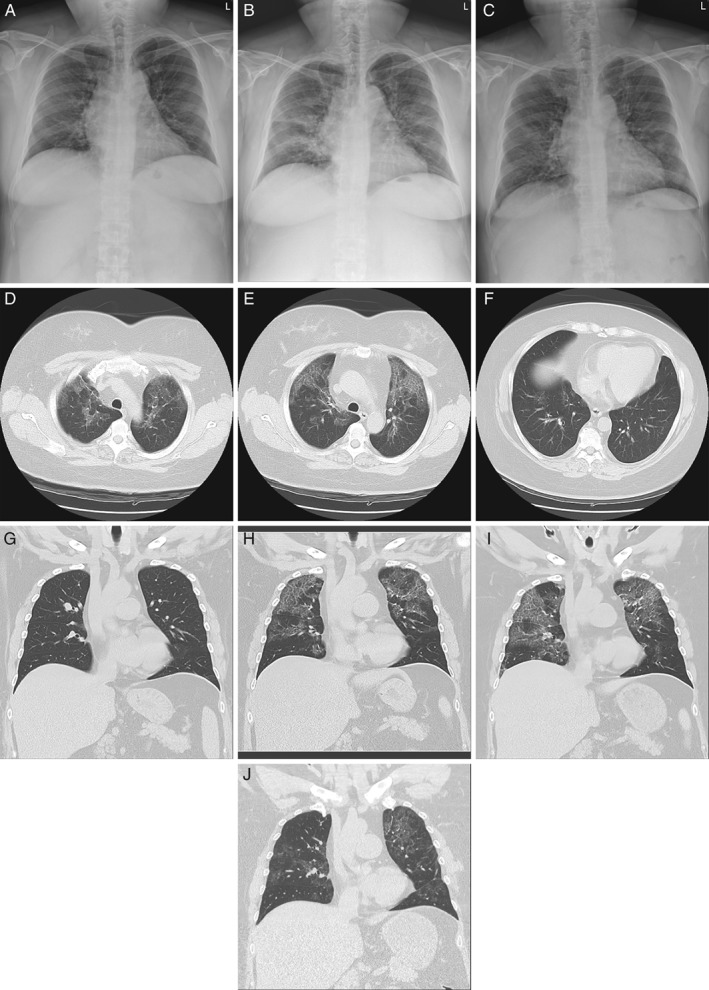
Serial radiographic images of the patient. Postero‐anterior chest roentgenograms taken one year earlier (A), at initial presentation (B), and 17 months later (C); computed tomography scan images: transverse sections at different levels (D–F) at initial presentation, and coronal views one year earlier (G), at initial presentation (H), six months later (I), and 17 months later (J).
